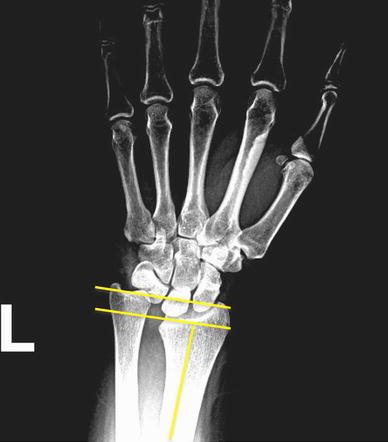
PA wrist radiograph: patient with a middle third radial shaft fracture, 4.27 mm ulnar positive with positive DRUJ instability. Ulnar variance measured with the digital calipers on the AP radiograph
